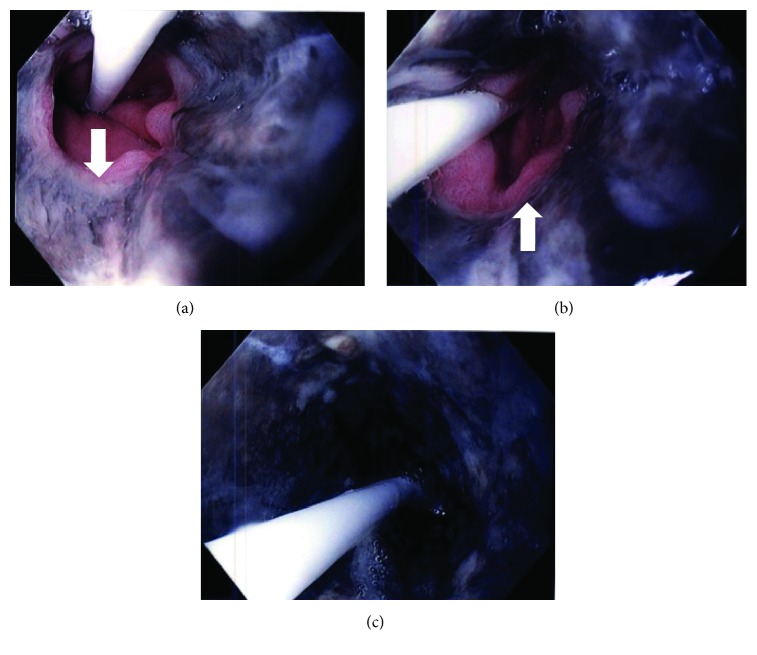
Images demonstrate circumferential esophageal necrosis extending from the mid esophagus (LL) to the distal esophagus with clear demarcation at the gastroesophageal junction (UL and UR with arrow). A transesophageal pressure catheter is seen in the images with distal end in the stomach.
